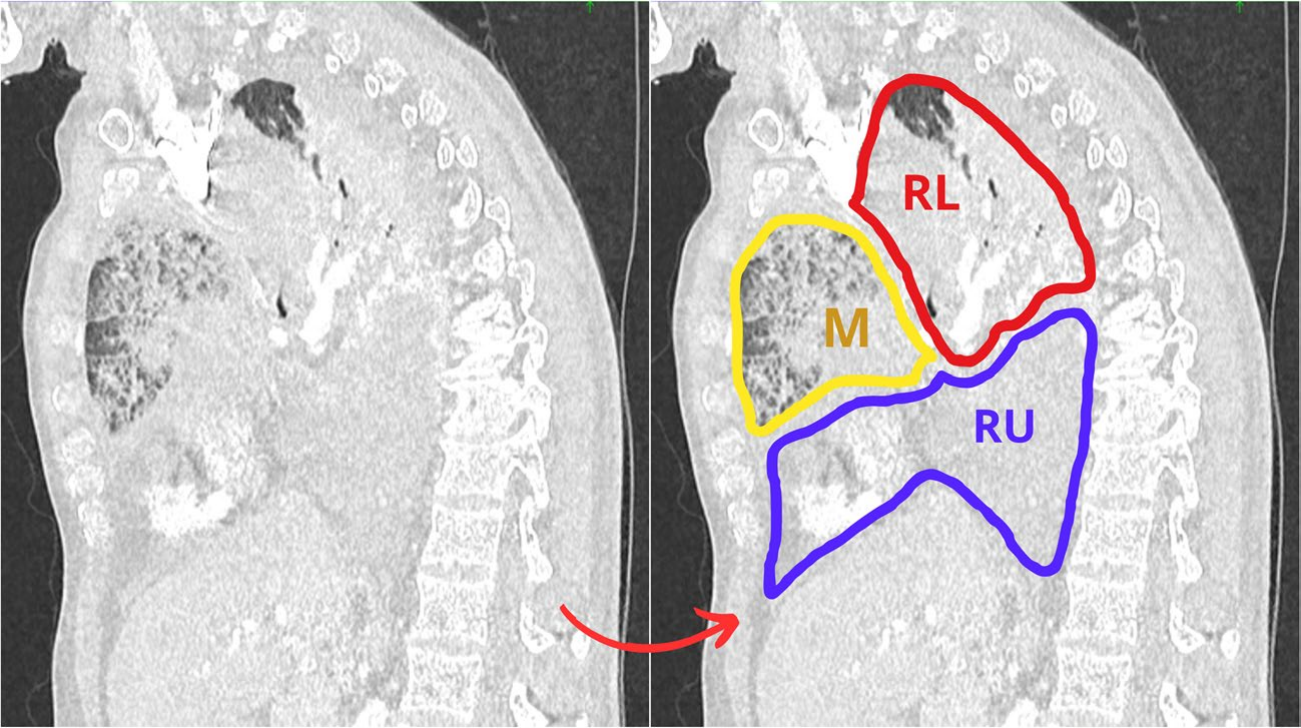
Preoperative computed tomography (CT) scan. The right upper (RU) lobe is in a lower position and completely necrotic (condensation, and haemorrhagic transformation). The middle (M) lobe is also necrotic in an anterior position (ground glass opacities). Both did not have any venous drainage or aeration. The right lower (RL) lobe is in an upper position with ventilated parenchyma remaining in the apex and effective venous drainage
